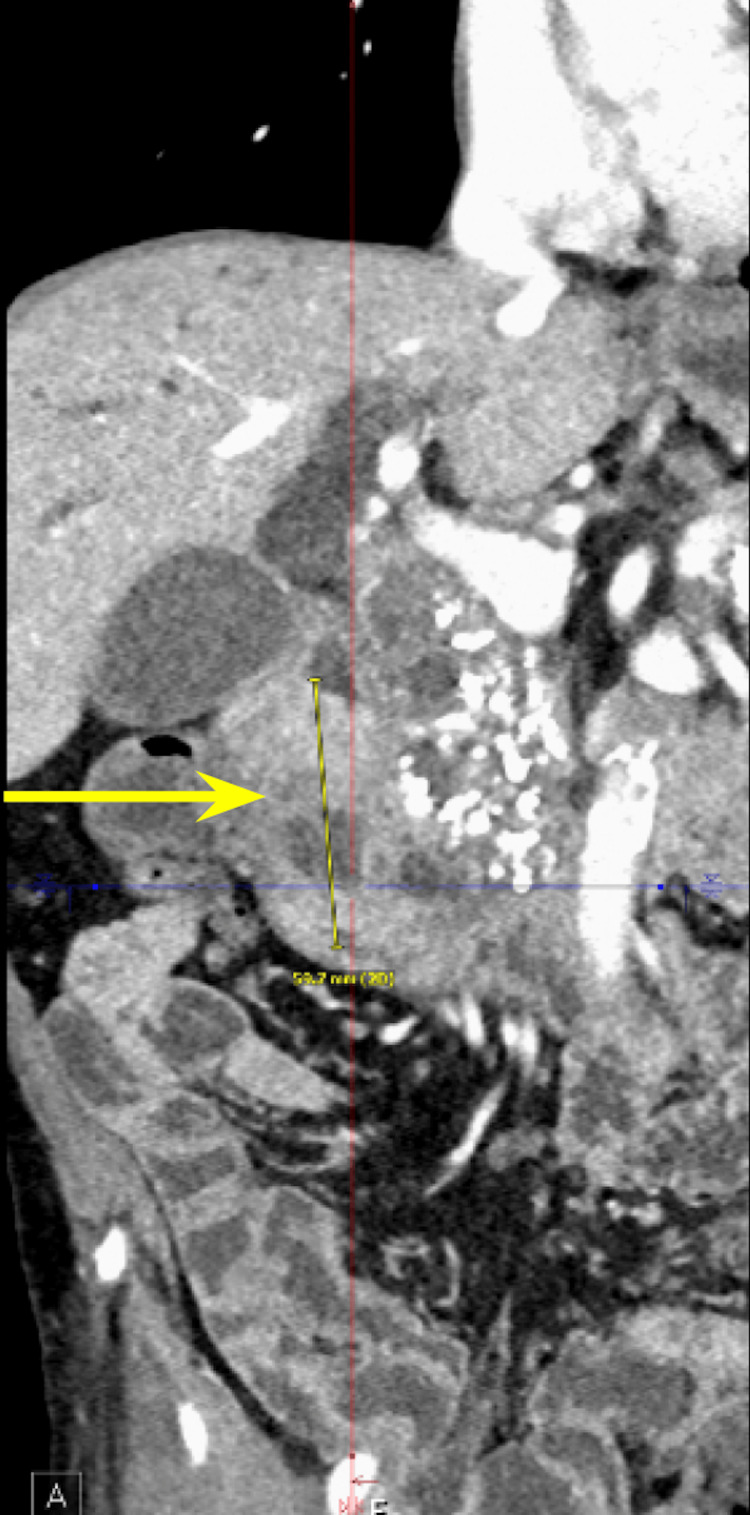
Computed tomography of the abdomen and pelvis showing a pancreatic mass measuring grossly 5.6 cm in the coronal plane.
